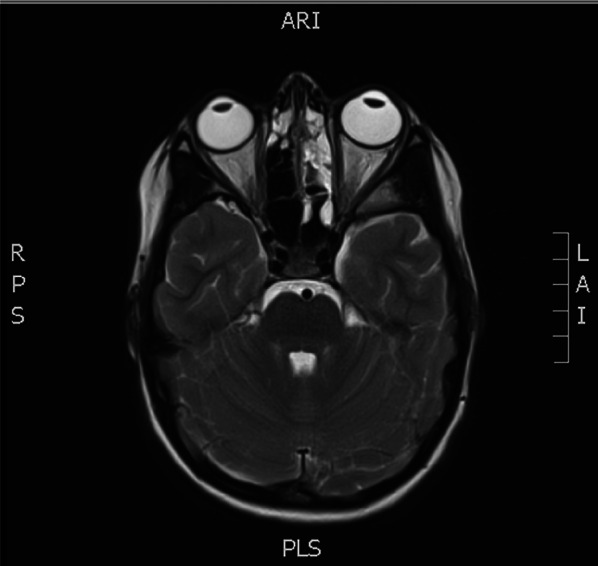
Patients MRI scan demonstrating paranasal sinusitis with near complete opacification of the left frontal, ethmoidal and maxillary sinuses and further mucosal thickening in the left posterior ethmoidal and sphenoidal sinus
